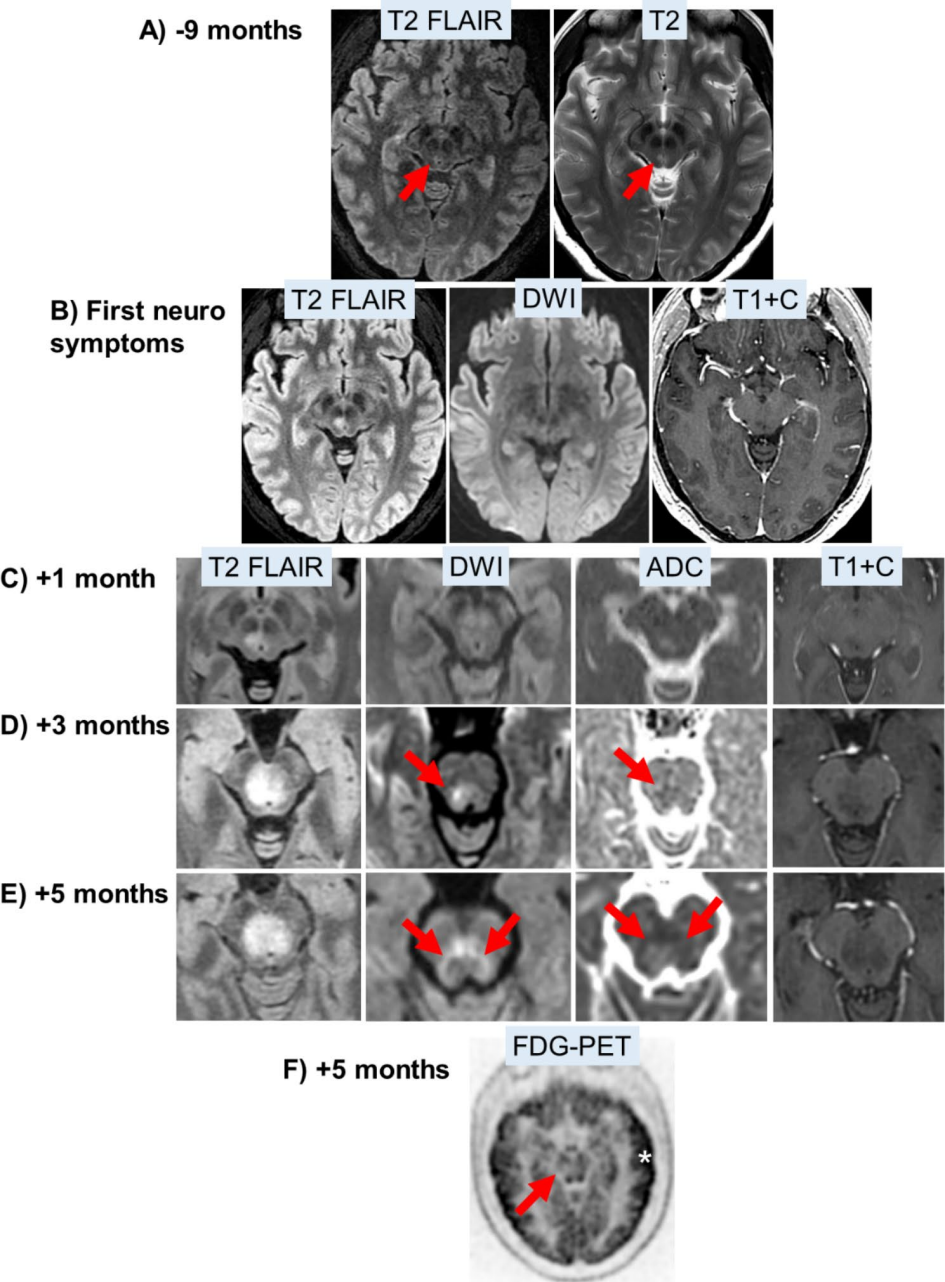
Radiologic progression of midbrain lesion. (A) Subtle T2/FLAIR-hyperintense lesion (red arrow) seen in retrospect 9 months prior to presentation with neurologic symptoms. (B) Right paramedian midbrain lesion at onset of neurologic symptoms, without contrast enhancement or diffusion restriction. (C-E) Growth of the lesion over 5 months, with gradual development of peripheral diffusion restriction (red arrows) but still no contrast enhancement. (F) FDG-PET shows midbrain lesion (red arrow) is hypometabolic compared to grey matter (*).
